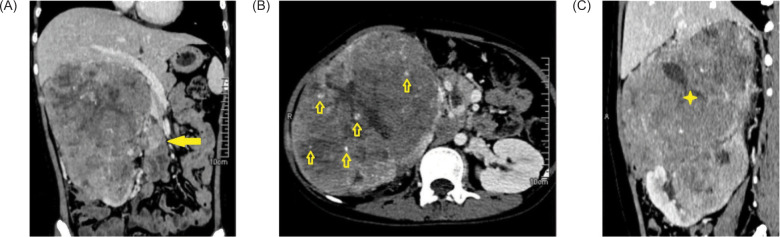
Contrast-enhanced CT-scan of the whole abdomen. (A) Renal mass with renal vein thrombosis (arrow). (B) Intratumoral calcifications (arrows). (C) Large heterogeneously-enhancing mass (star) arising from the kidney.
